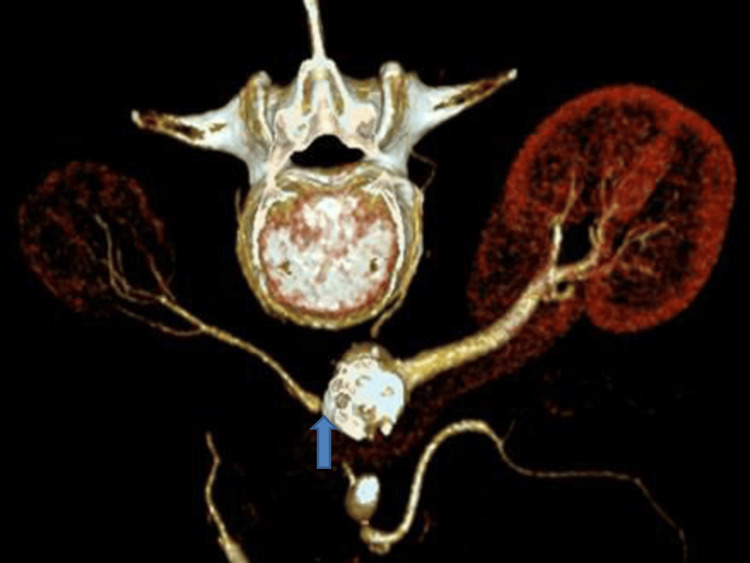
3D reconstruction of abdominal and pelvic axial CT scans. The arrow indicates the origin of the right renal artery, with significant aortic atherosclerosis and consequent atrophy of the ipsilateral kidney.
